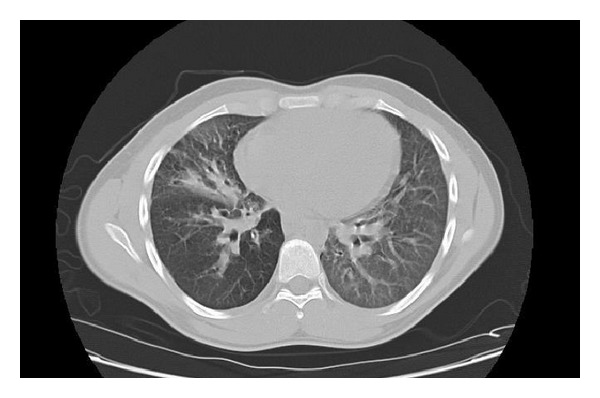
CT scan of the chest showing central bronchiectasis.
